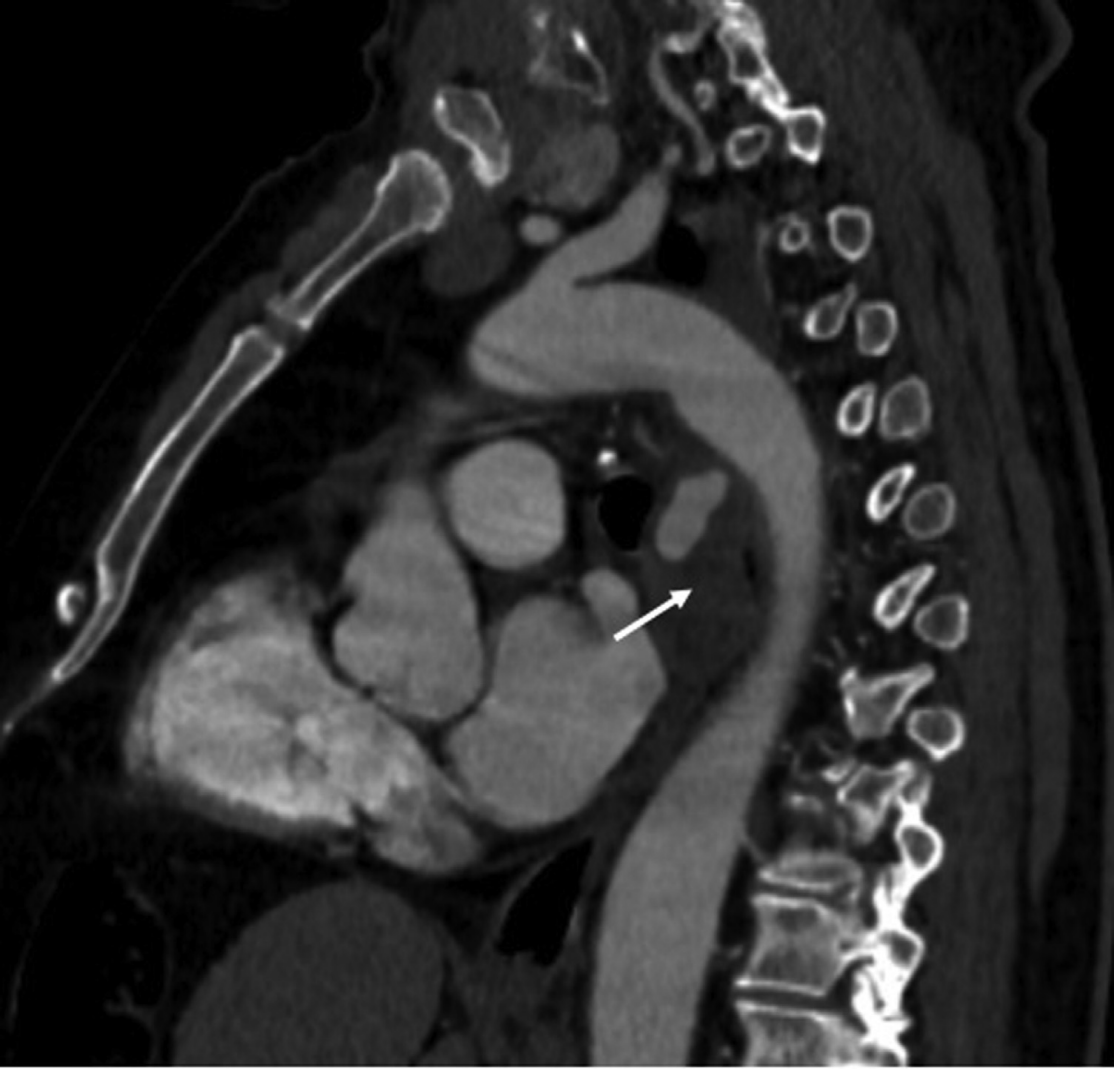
sagittal CT scan view in the arterial phase showing a mediastinal partially thrombosed aneurysm (arrow). There was no evidence of communication with the descending aorta excluding the hypothesis of an aortic aneurysm.
